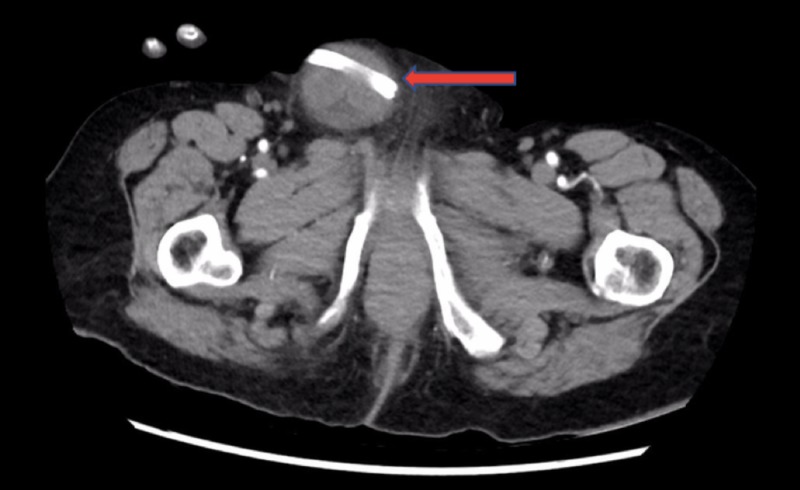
Abdominal CT axial view demonstrating a pair of biliary stents (red arrow) within a bowel containing right inguinal hernia.
